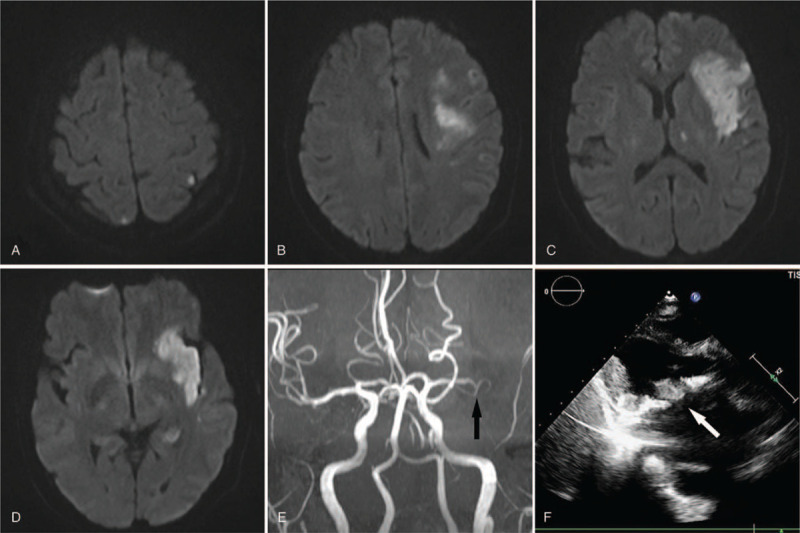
Cranial MRI. Axial diffusion-weighted imaging (DWI) showed high-signal lesions comprising the left frontal-temporal-parietal lobe, insula, hippocampus and thalamus as well as the right parietal lobe (A–D), with low apparent diffusion coefficient signals in the corresponding regions (not shown), and MR angiography (MRA) indicated the left middle cerebral artery occlusion (E, black arrows). Echocardiogram. Echocardiogram suggested the mural thrombus formation in the anterior leaflet of the mitral valve (F, white arrows). MRI = magnetic resonance imaging.
